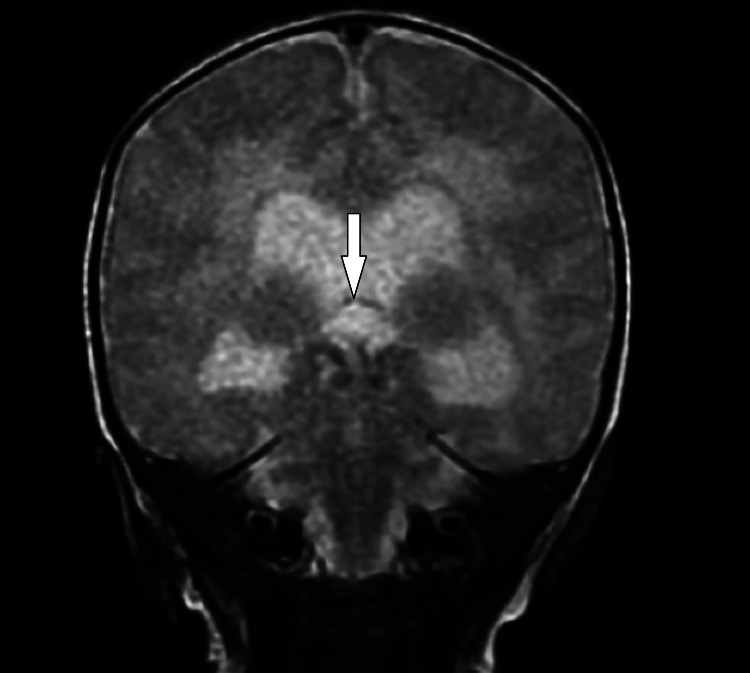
Coronal view MRI T2WI coronal view shows fused cerebellar hemispheres across the midline with the absence of vermis accentuated by the 'Donald Duck' sign with dilated lateral and third ventricle secondary to aqueductal stenosis T2WI: T2 weighted image
